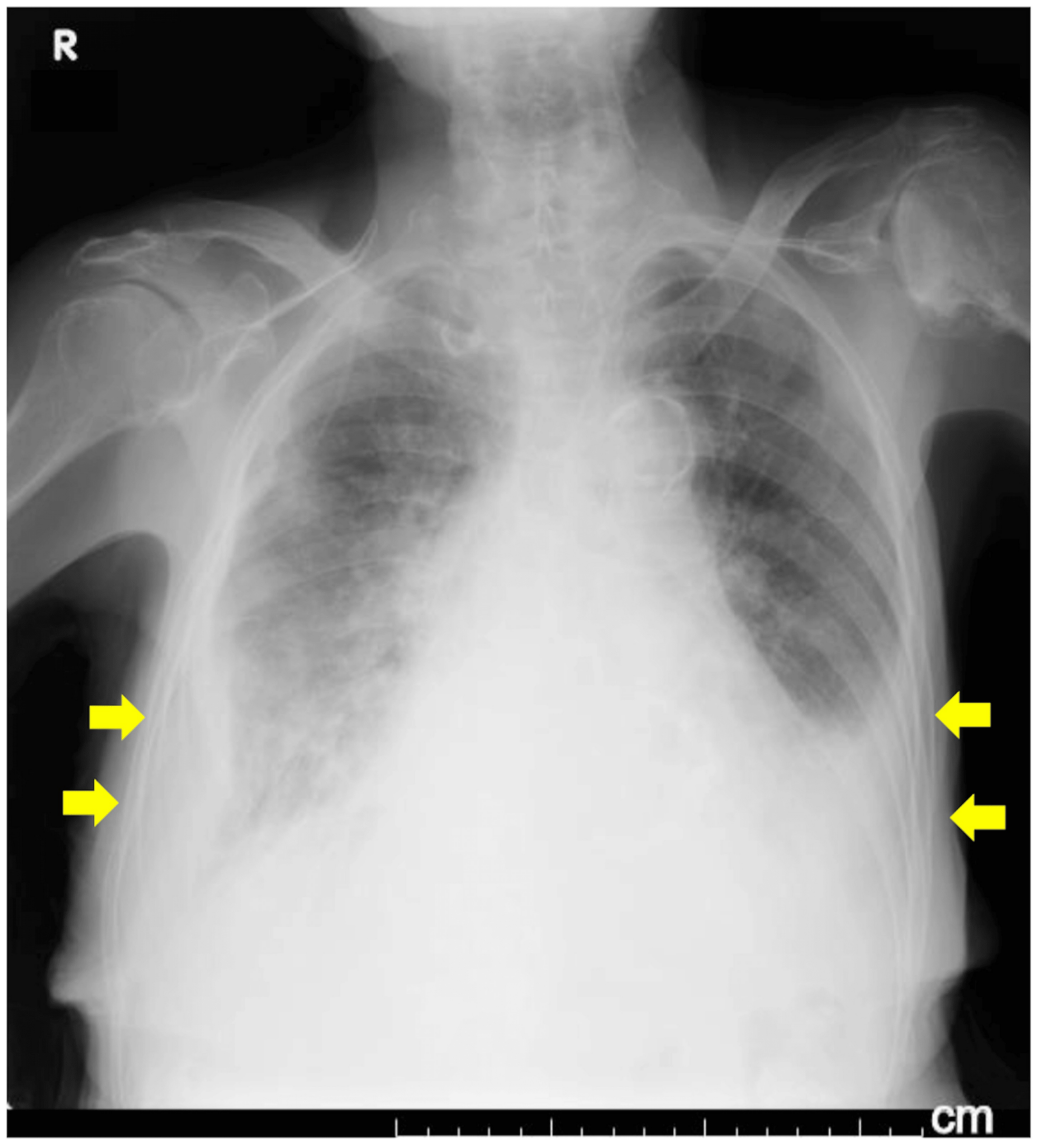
Chest radiography on admission showing cardiomegaly with pulmonary congestion and pleural effusion (arrows) NOTE: Cardiothoracic ratio could not be accurately measured
